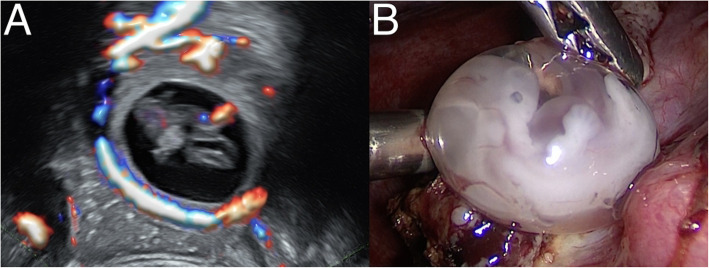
A Sonographic appearance of an ectopic pregnancy showing the ‘ring of fire’ sign and an embryonic cardiac signal. B Laparoscopically extracted embryonic sac
